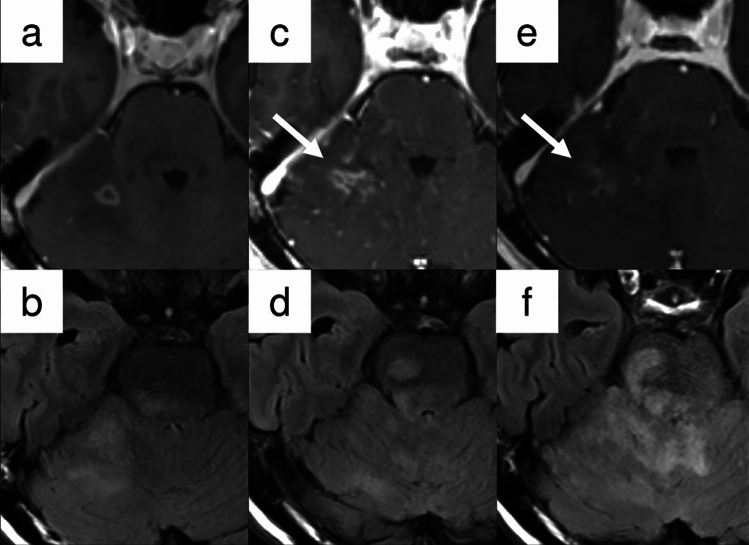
Case 6: A 75-year-old man presented with a tumor involving the cerebellum and pons. Stereotactic biopsy suggested diffuse midline glioma with H3K27-altered status (a and b). Eight months following completion of concurrent CRT, surveillance imaging revealed increased contrast-enhancing foci (arrow), raising concern for disease progression (c and d). The patient was subsequently initiated on second-line chemotherapy including bevacizumab. Follow-up imaging at one month demonstrated rapid resolution of contrast enhancement (arrow) (e) as well as progression of T2-FLAIR lesion (f); consistent with pseudoresponse and non-enhancing tumor progression, a characteristic imaging pattern associated with anti-angiogenic therapy
